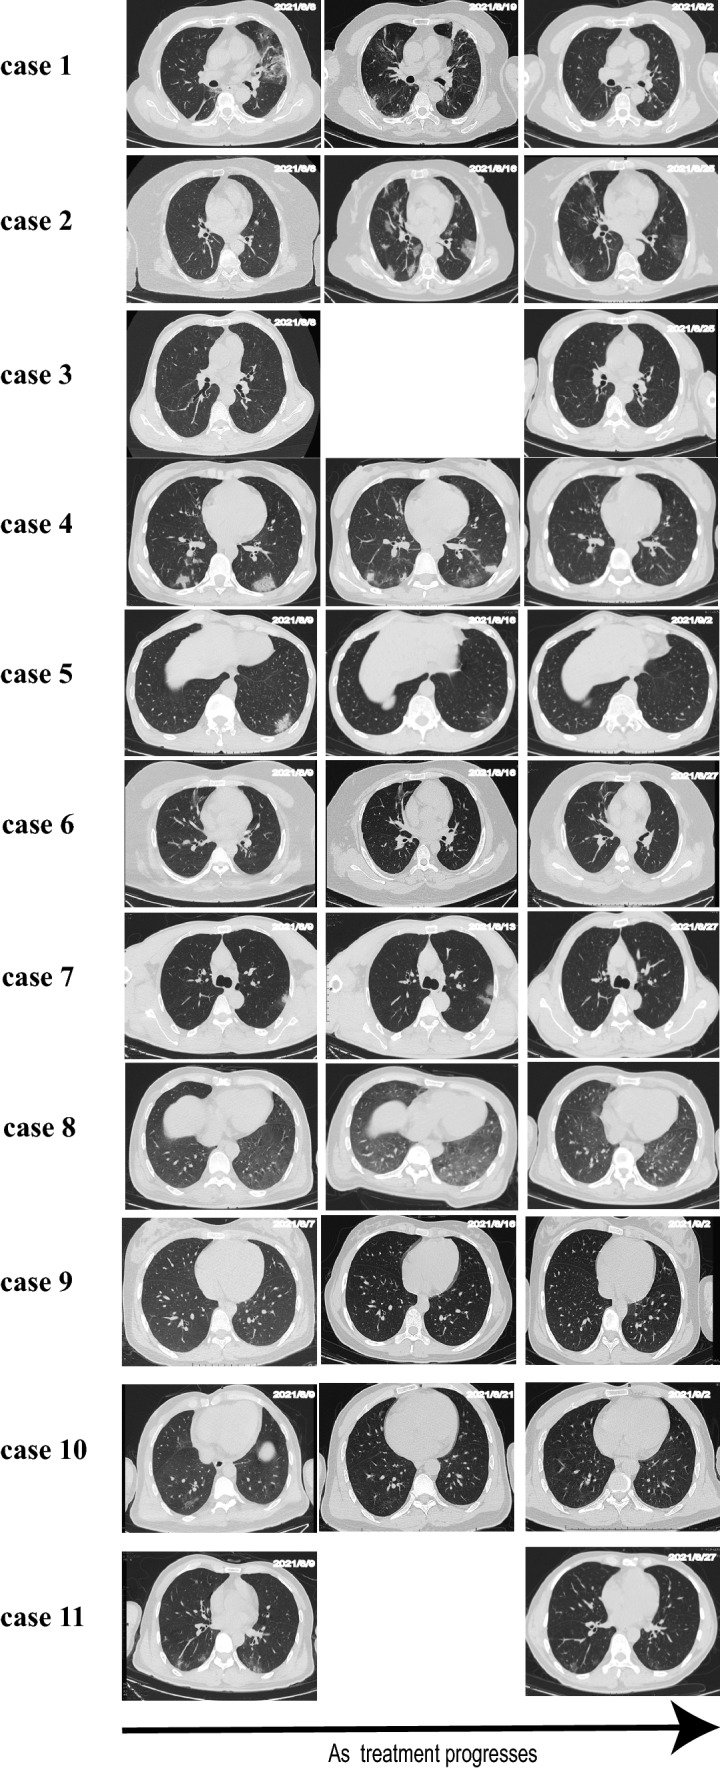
Evolution of the lesions on chest CT of patients with COVID-19/HIV co-infection. As treatment progresses, the lesions f CT images gradually disappear. CT of one case is absent
